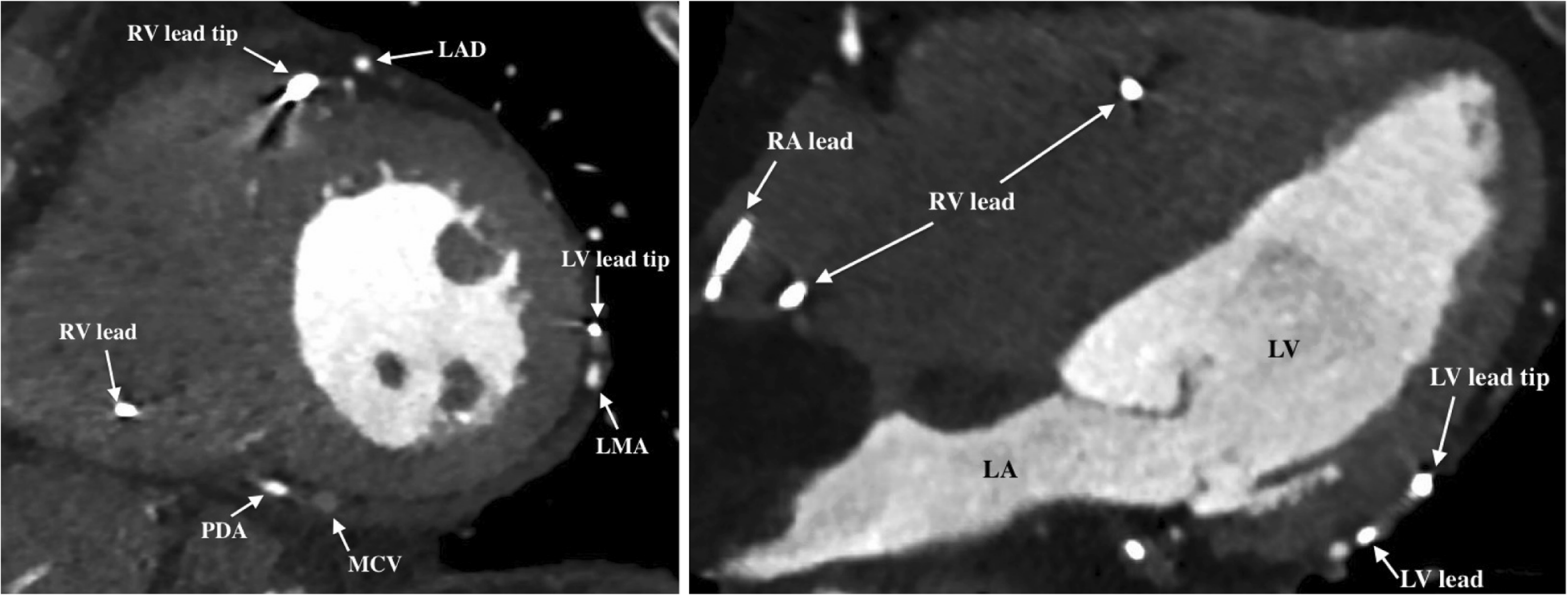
Evaluation of LV lead position. Six months follow-up cardiac CT acquisition from the same patient as in Figure 4. In the mid-LV short axis view, the LV lead is positioned in the left marginal vein draining the lateral LV wall (left). In the four-chamber view, the LV lead tip is positioned in the lateral mid-LV segment (right). LA, left atrium; LAD, left anterior descending artery; LMA, left marginal artery (circumflex artery branch); MCV, middle cardiac vein; PDA, posterior descending artery (right coronary artery branch); RA, right atrium; RV, right ventricle).
